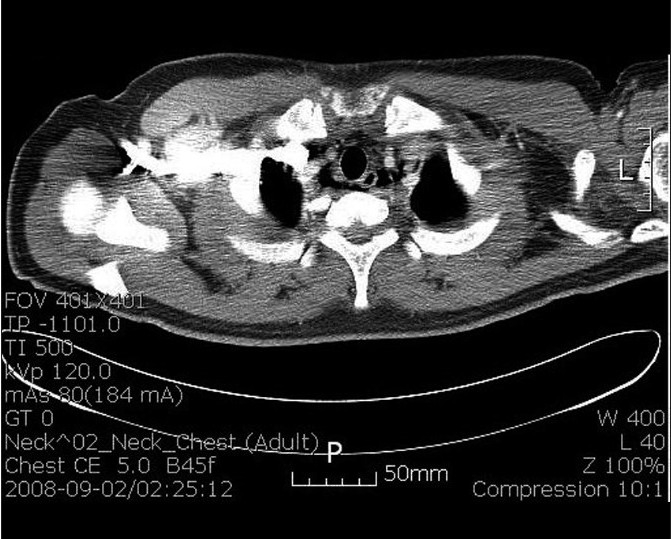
Chest CT scan of case 1 reveals pneumomediastinum
